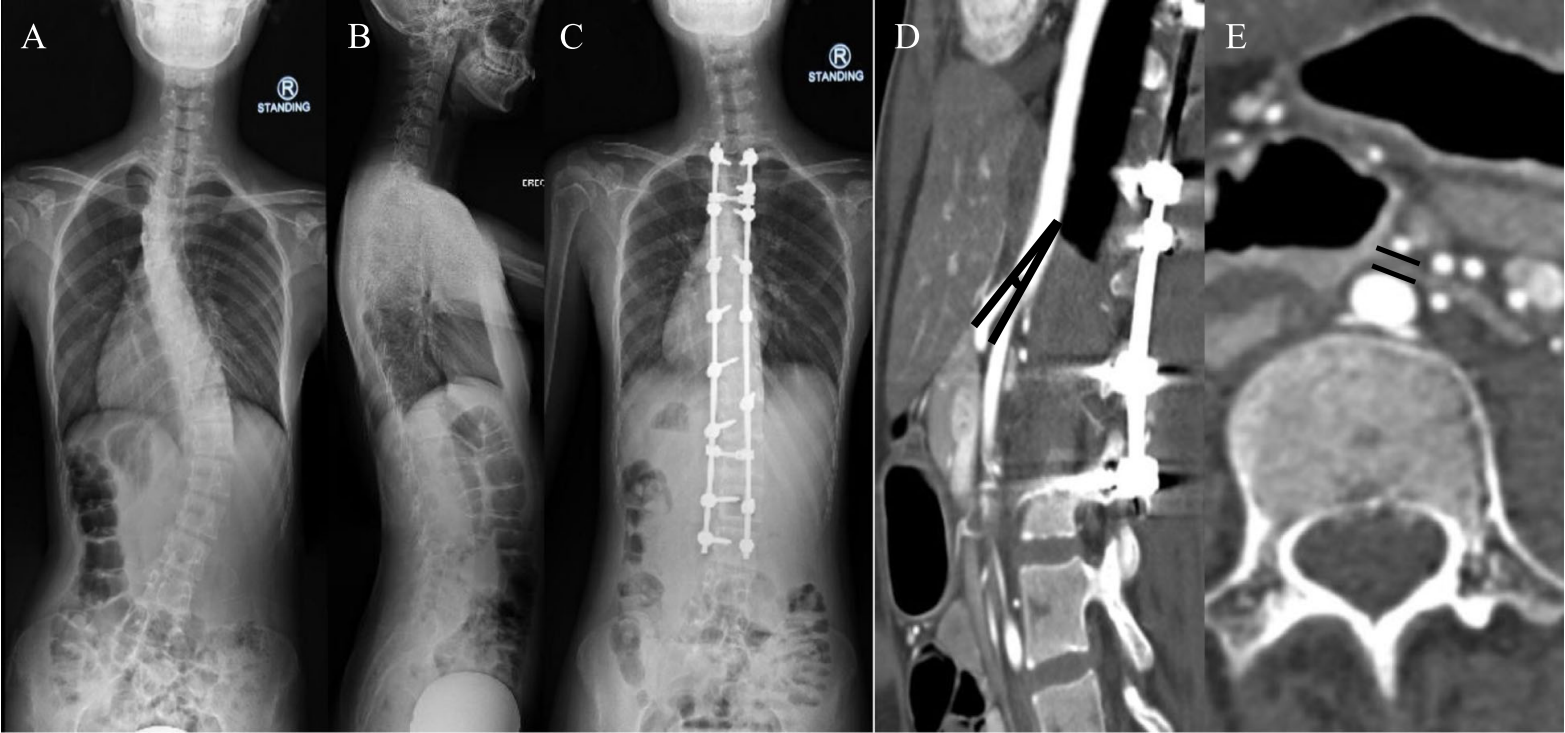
One case with superior mesenteric artery (SMA) syndrome after scoliosis surgery (preoperative x-ray – a & b, postoperative AP x-ray – c). Sagittal (d) and axial (e) of computed tomography angiography (CTA) of the abdomen revealed aortomesenteric angle of 10°, SMA-aorta distance of 3.2 mm and constriction of 3rd part of duodenum by the branches of the SMA
